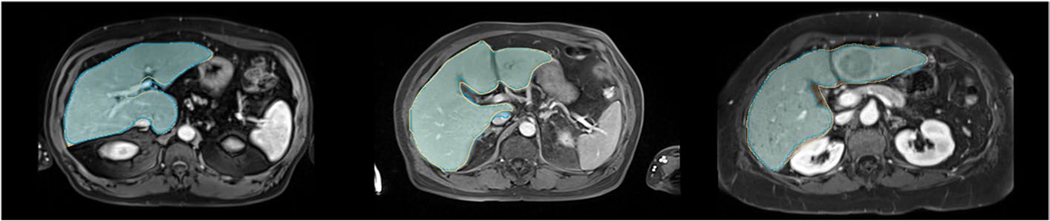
Representative segmentations. Representative liver segmentations on axial portal venous phase contrast-enhanced magnetic resonance images from the internal (left), external (middle), and public (right) test sets with Dice similarity coefficients of 0.977, 0.964, and 0.944, respectively. The manual liver segmentations are overlaid in yellow, and the automated segmentations are overlaid in blue
